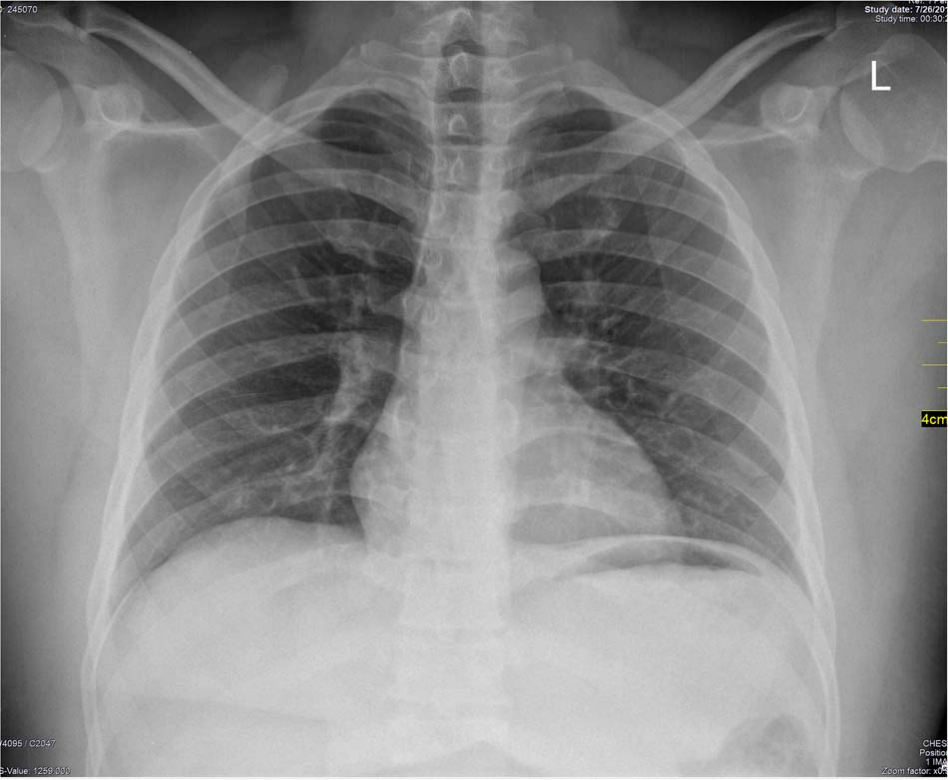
Chest X-ray PA view; normal chest X-ray.
